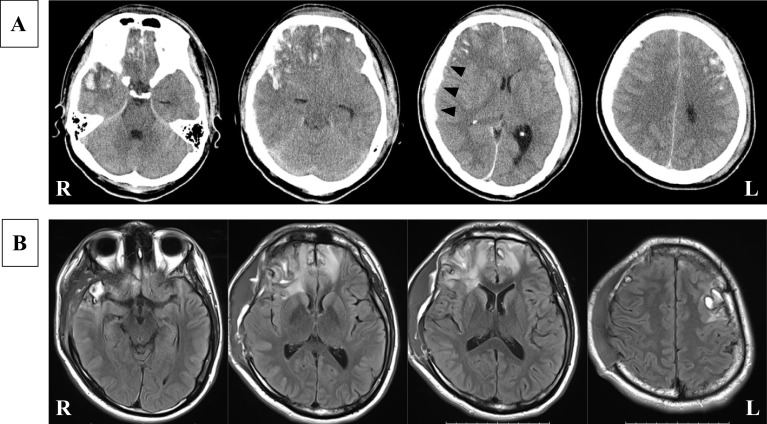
A Head CT scan on the day of injury showed multiple brain contusions (salt and paper appearance) in the right frontal and temporal lobes and left frontal lobe along with a right acute subdural hematoma (arrow heads). B FLAIR MR imaging 23 days after the injury showed multiple high-intensity regions in the right frontal and temporal lobes and left frontal lobe along with high-intensity areas in the white matter surrounding the brain contusions
